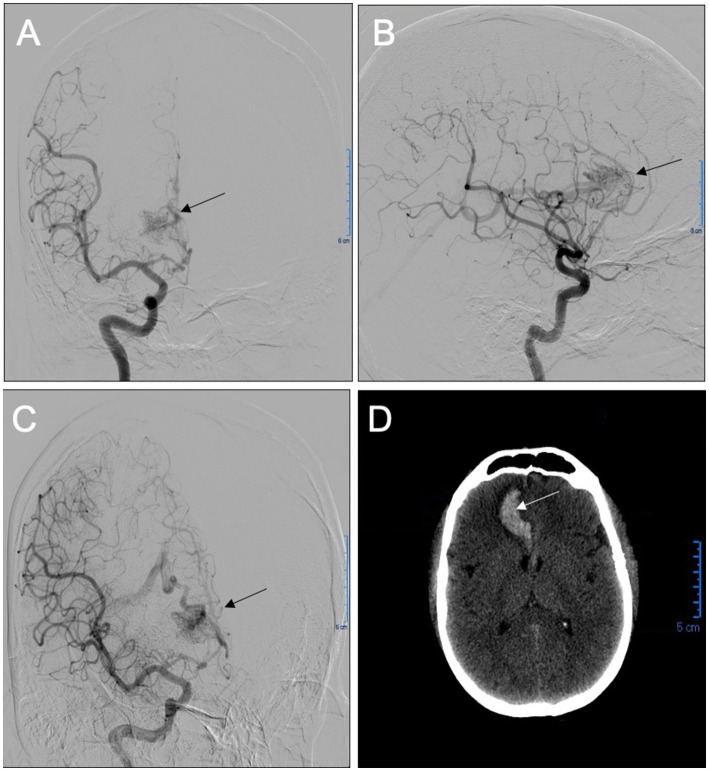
Anteroposterior (A), lateral (B), and oblique (C) projections of a preoperative digital subtraction angiography (DSA) from a right internal carotid artery injection demonstrated a diffuse right frontal arteriovenous malformation (AVM), with feeders from the right anterior cerebral artery and deep venous drainage. Preoperative head CT (D) shows an intraparenchymal hematoma centered in the right medial frontal lobe extending to the callosal sulcus.
